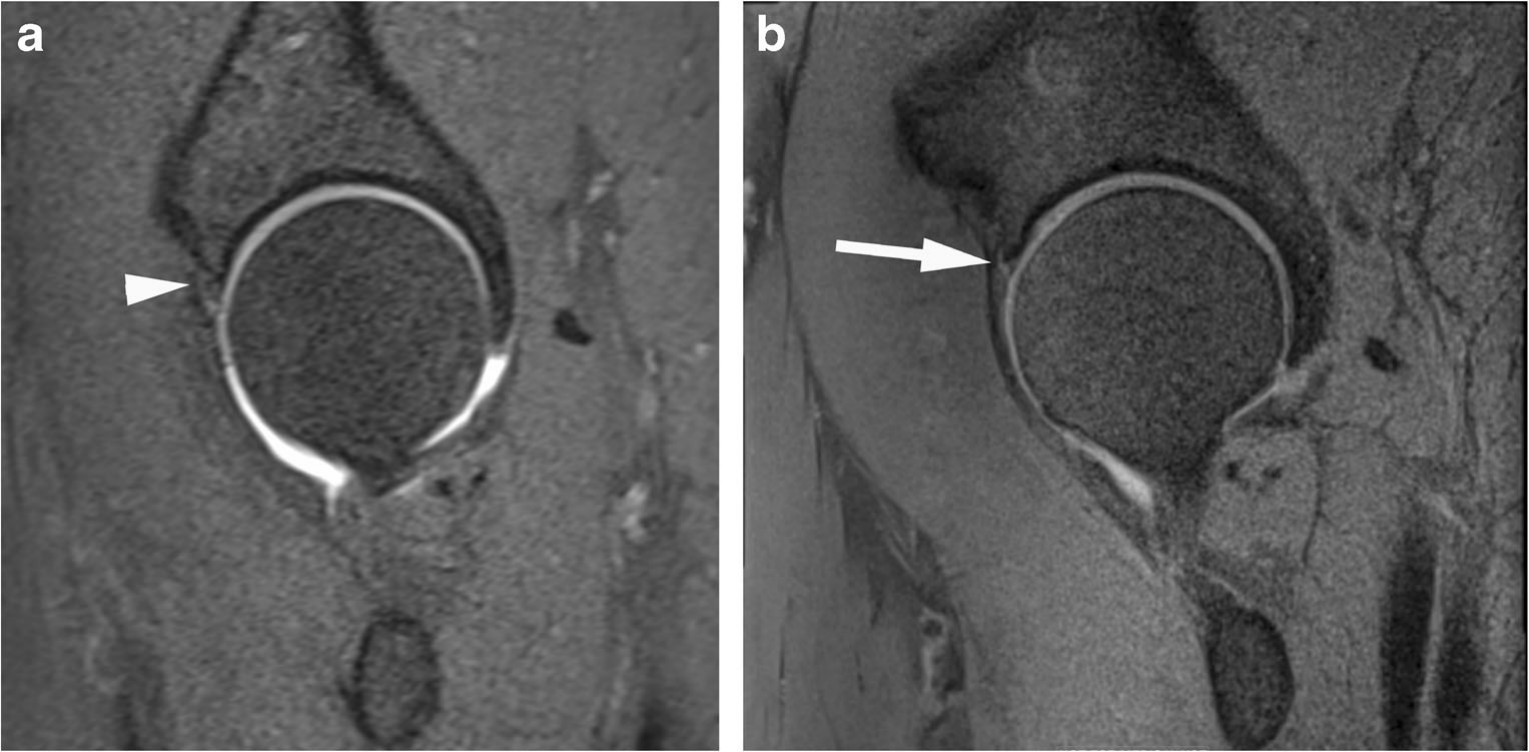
Acetabular labrum complete tear in a 29-year-old confirmed at surgery. Sagittal (a) T1 FS 1.5T MRA shows no tear (arrowhead) and (b) PD FS 3T MR images shows complete basal labral tear (arrow)
